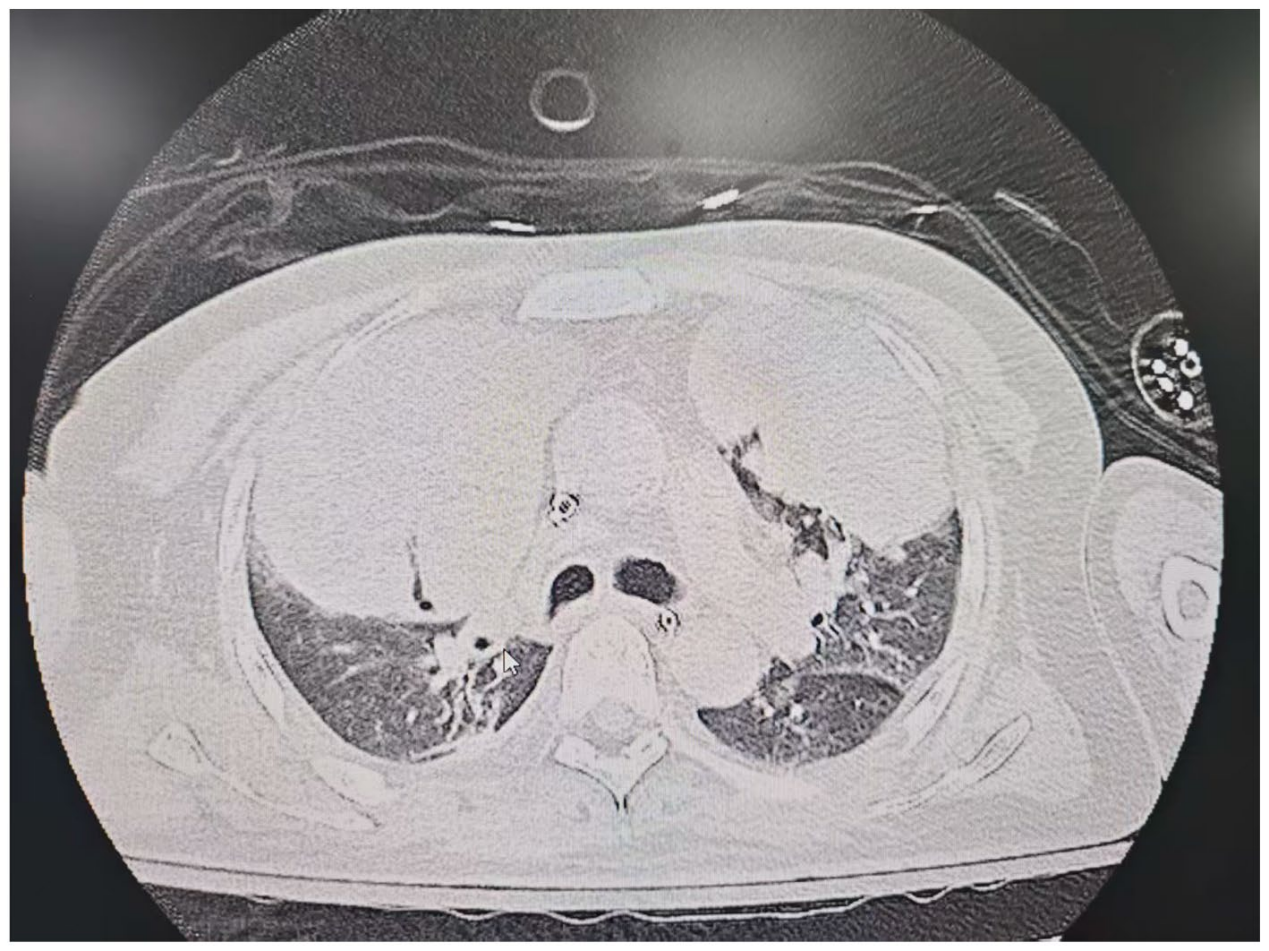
Lung CT on the day of ECMO weaning.
